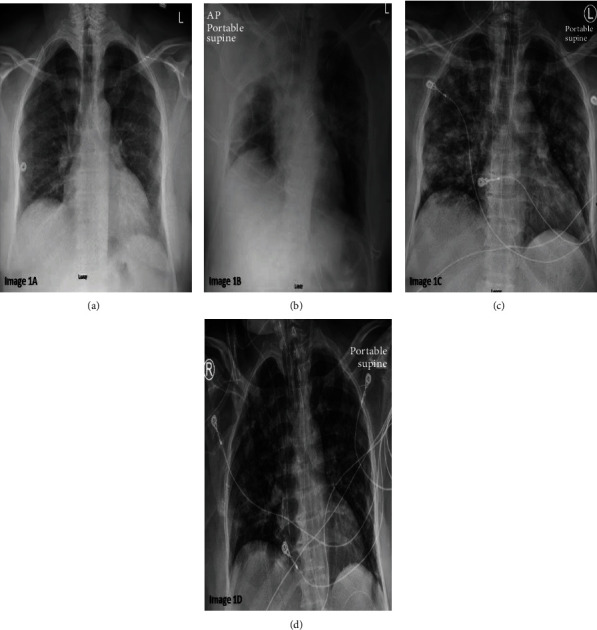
(a) Chest X-ray, PA view on initial presentation to emergency room showing increased interstitial markings bilaterally. (b) Chest X-ray, AP view done on patient's second emergency room visit showing bilateral patchy consolidations and worse in right lung fields. (c) Chest X-ray, PA view on hospital day 3 showing persistent bilateral patchy consolidations improved from hospital day 1. (d) Chest X-ray, PA view on hospital day 7 showing improved bilateral consolidations.
